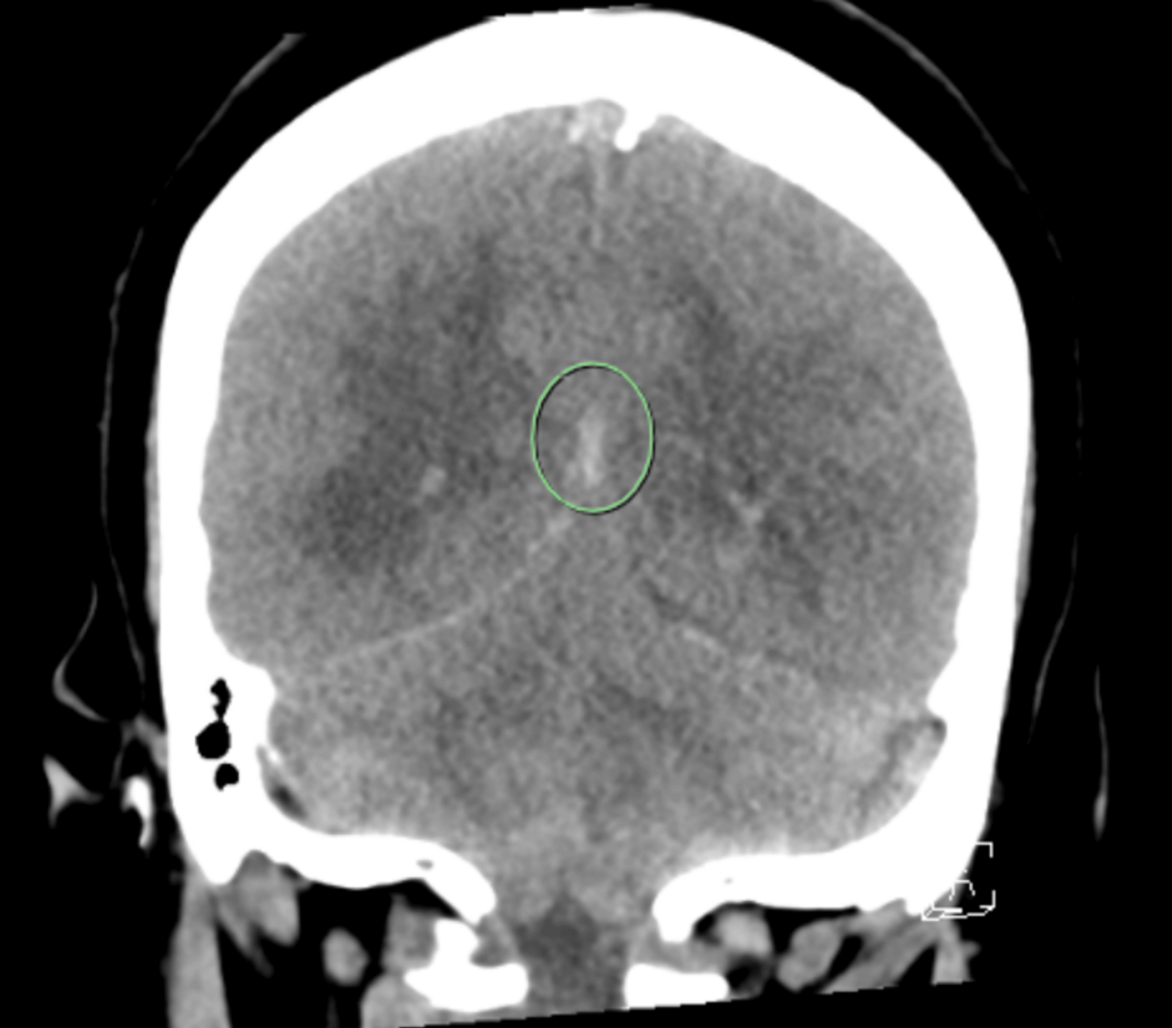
Coronal View of Head CT Imaging demonstrating subarachnoid hemorrhage.
